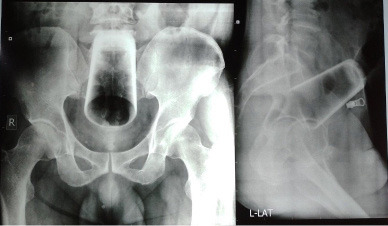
X-ray abdomen erect-AP and lateral.
